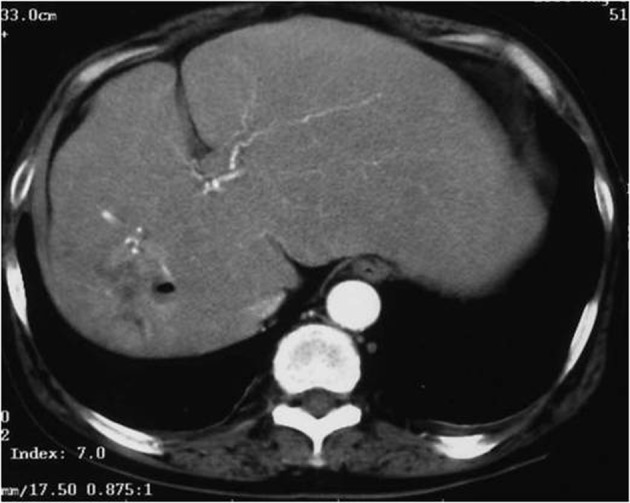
Computed tomography with contrast medium (arterial phase).
